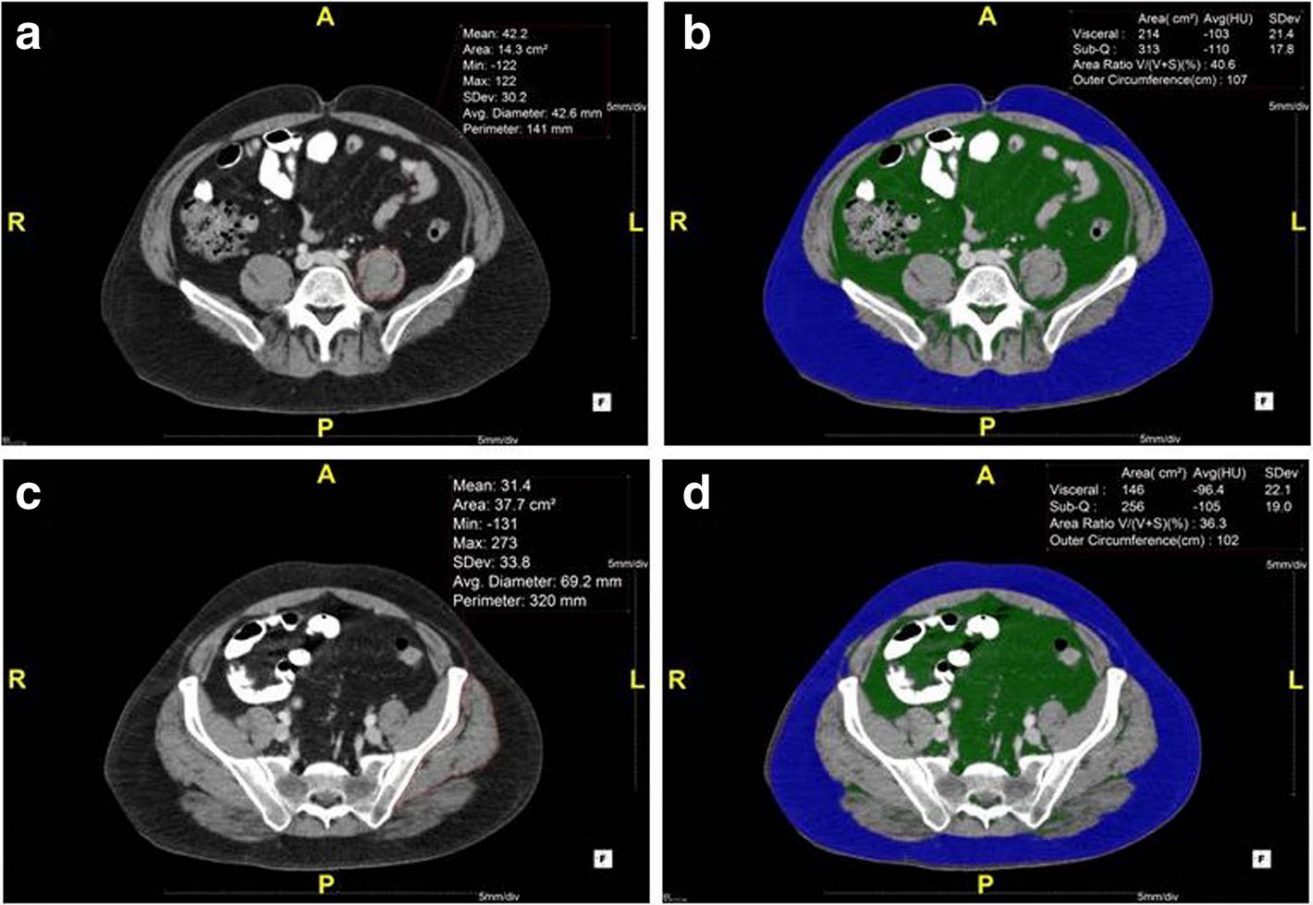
Representations of the CT slices analyzed: (a) psoas slice with muscle polygon, (b) psoas slice with fat analysis algorithm, (c) gluteal slice with muscle polygon, and (d) gluteal slice with fat analysis algorithm
